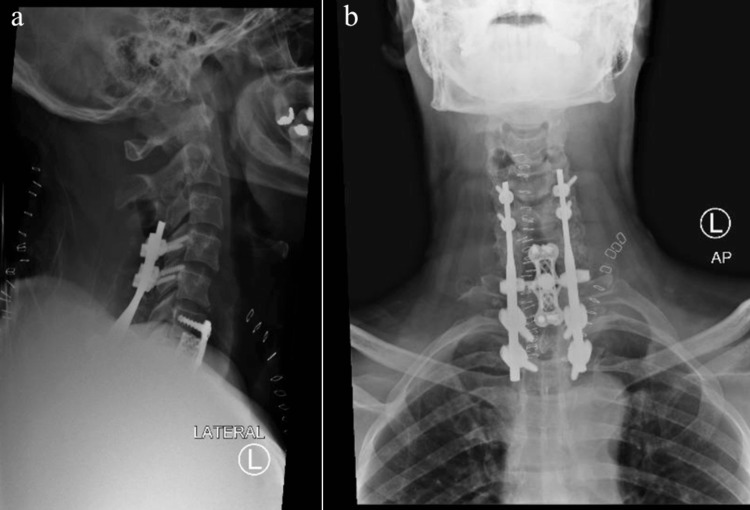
Postoperative anterior-posterior and lateral X-ray images. Immediate postoperative plain radiographs showed good realignment and correct screw placements
